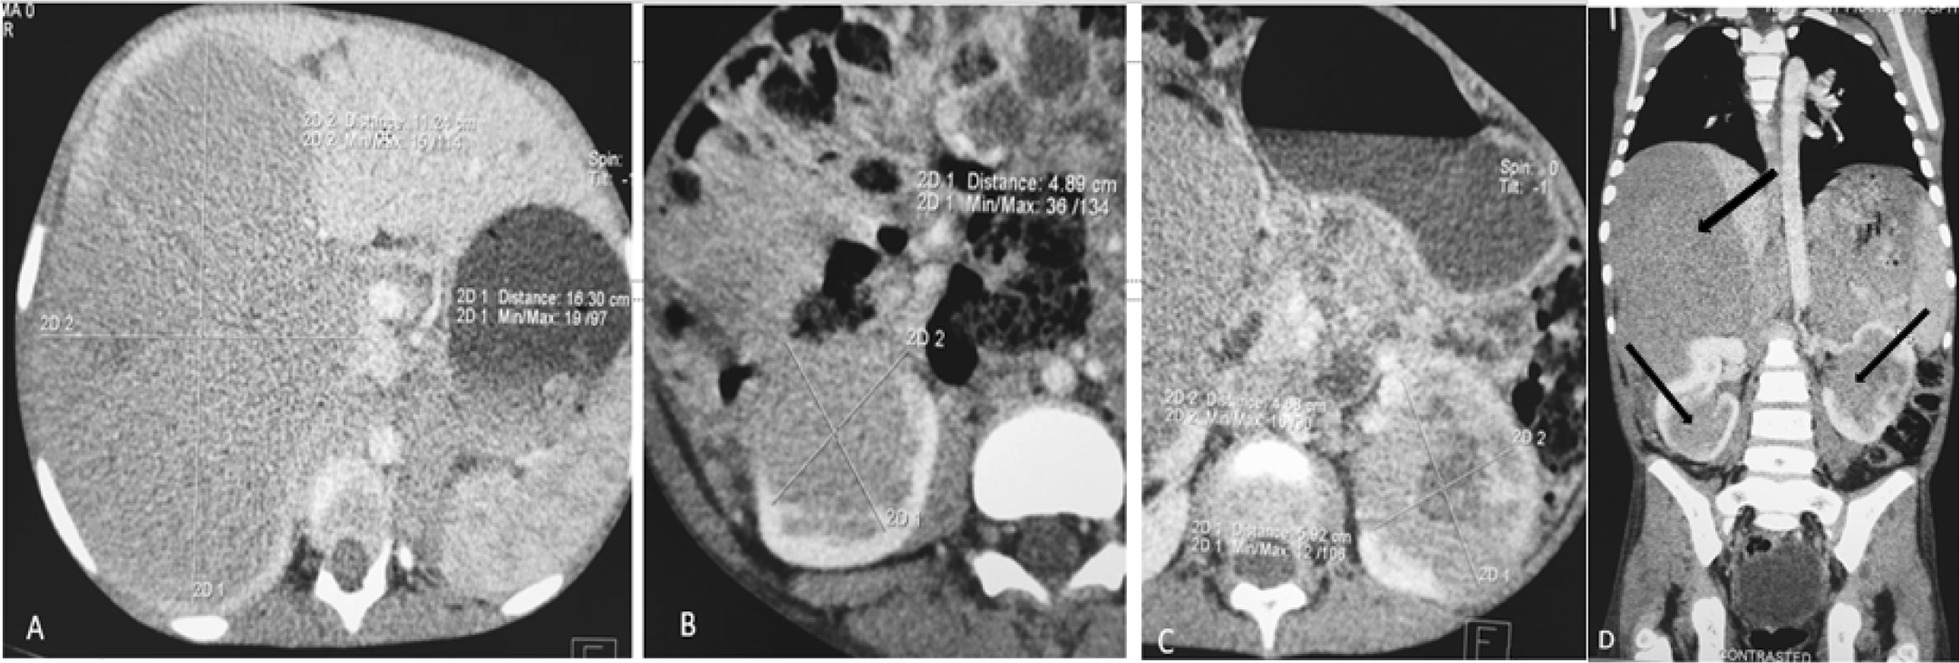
Contrasted CT scan of the abdomen axial (A–C) and chest, abdomen, pelvis coronal plane (D). A Homogenously enhancing (35–62 HU) hypo dense solid mass in the segments V, VI, VII and VIII; B heterogeneously hypo dense solid mass in the right kidney involving middle to inferior pole; (C) hypo dense solid mass with central necrosis involving the whole left kidney; D coronal plane of the chest, abdomen, pelvis on soft tissue window demonstrated masses in the liver, right kidney (sparing small portion of the superior pole), left kidney
